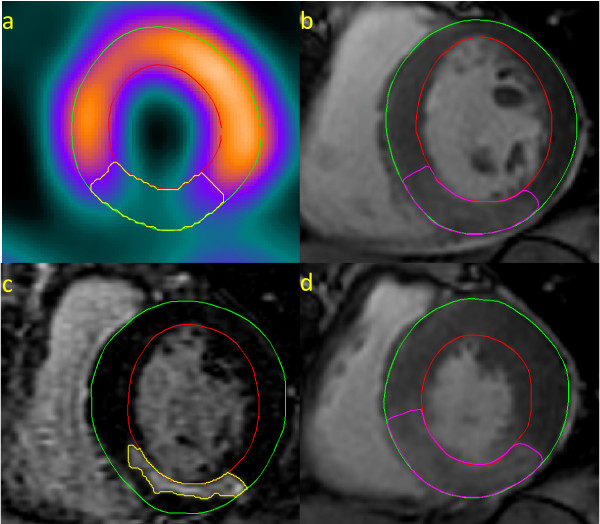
Inferior STEMI. Corresponding left ventricular short axis views from a patient with inferior STEMI. MaR determined by (a) myocardial perfusion SPECT, (b) gadolinium enhanced SSFP at end-diastole, (c) infarct size images with LGE and (d) gadolinium enhanced SSFP at end-systole.
